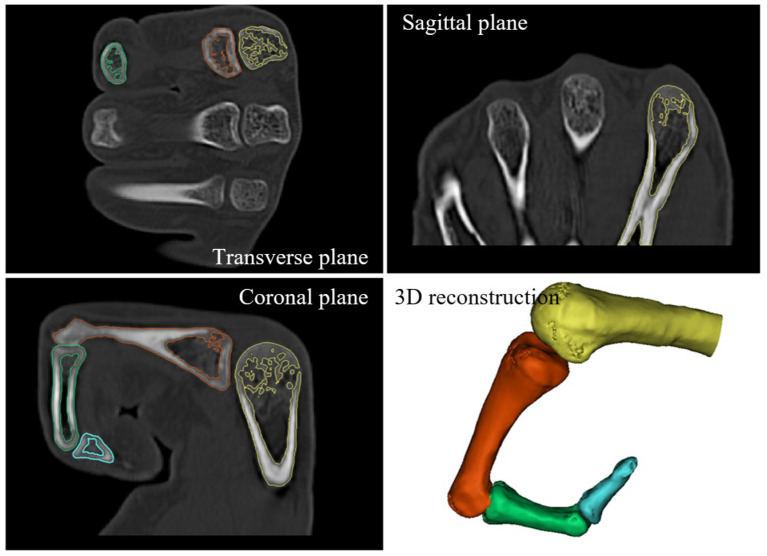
CT imaging processing results of index finger (The metacarpal bone is yellow, the proximal phalanx is orange, the middle phalanx is green, and the distal phalanx is blue).
